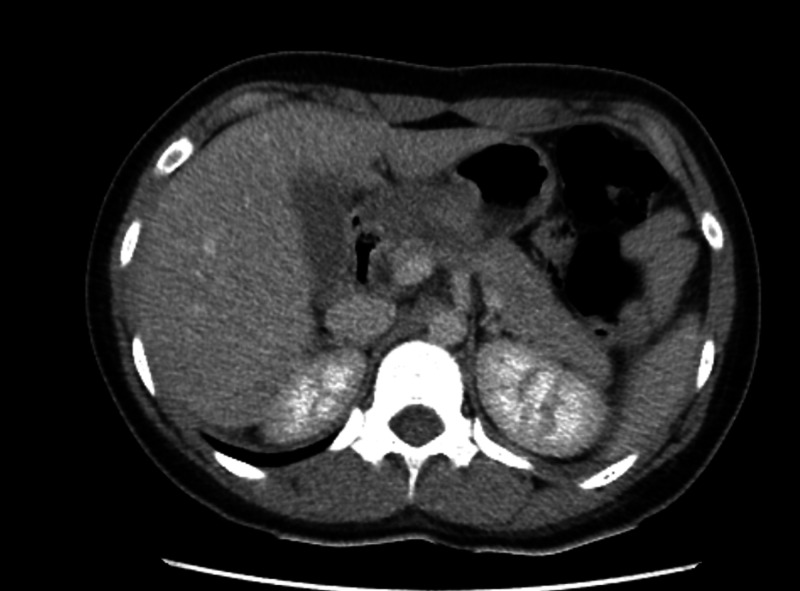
Normal CT image of the liver despite grossly abnormal liver function tests
